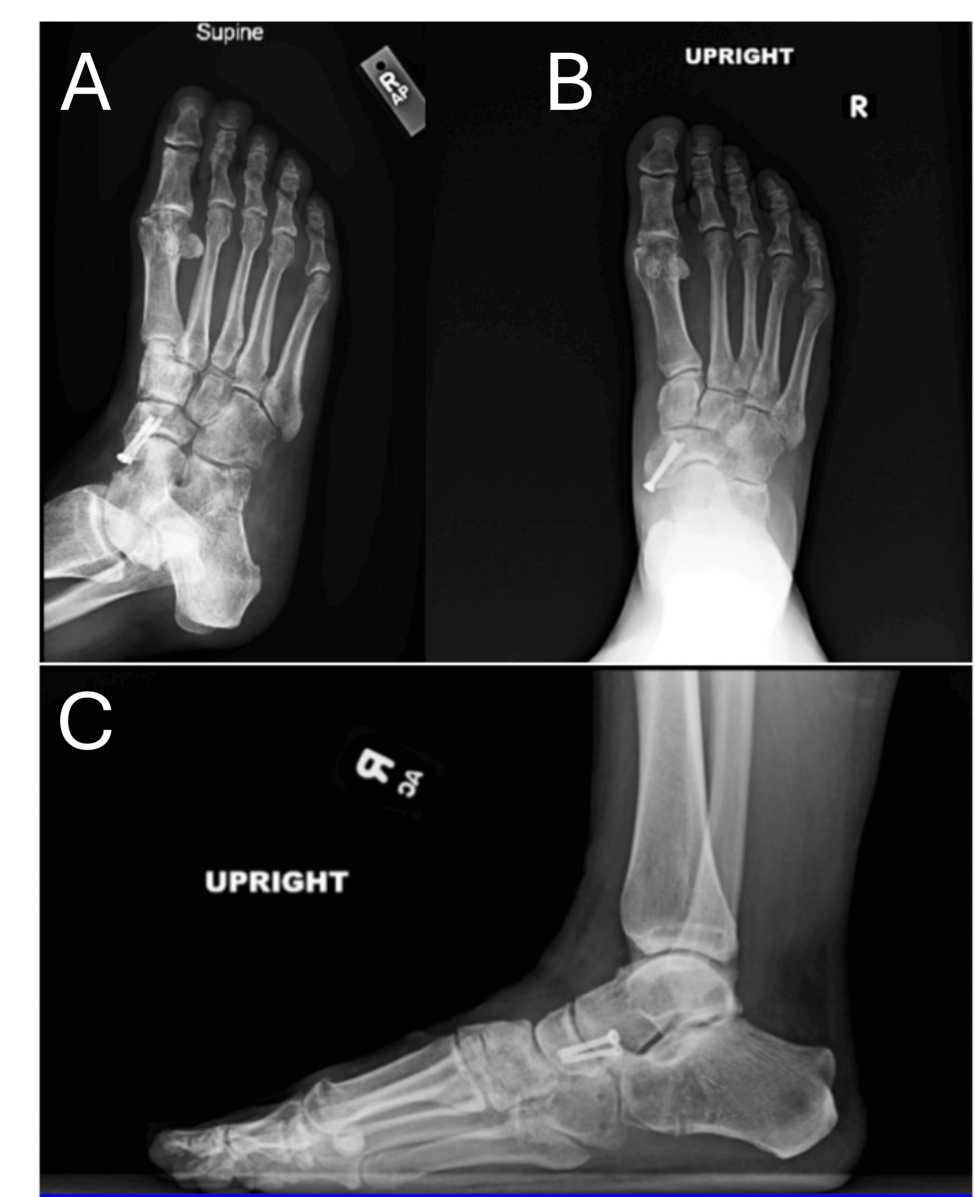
Standing AP (B), lateral (C), and oblique (A) radiographs obtained at four-month follow-up after elective removal of K-wire and spanning calcaneocuboid joint. K-wire, Kirschner wire
